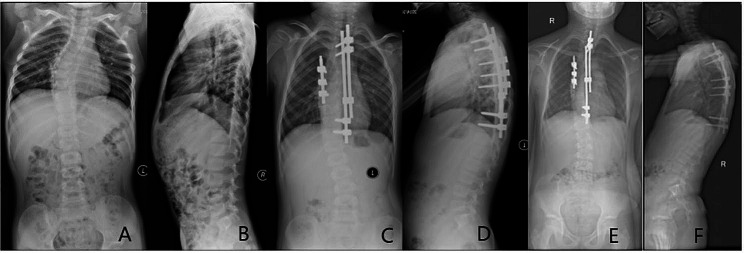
6-year-old boy, syndromic early onset scoliosis. A, B, Preoperative X-ray; C, D, use convex side block combined with concave side single growing rod technique, after first operation, X-ray; E, F, Sixty-eight months (four lengthening) follow up
